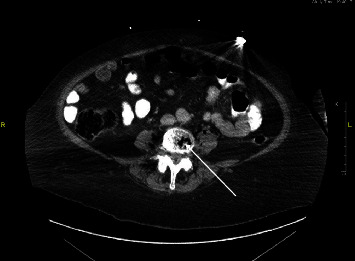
CT abdomen and pelvis showing pneumoracchis.
